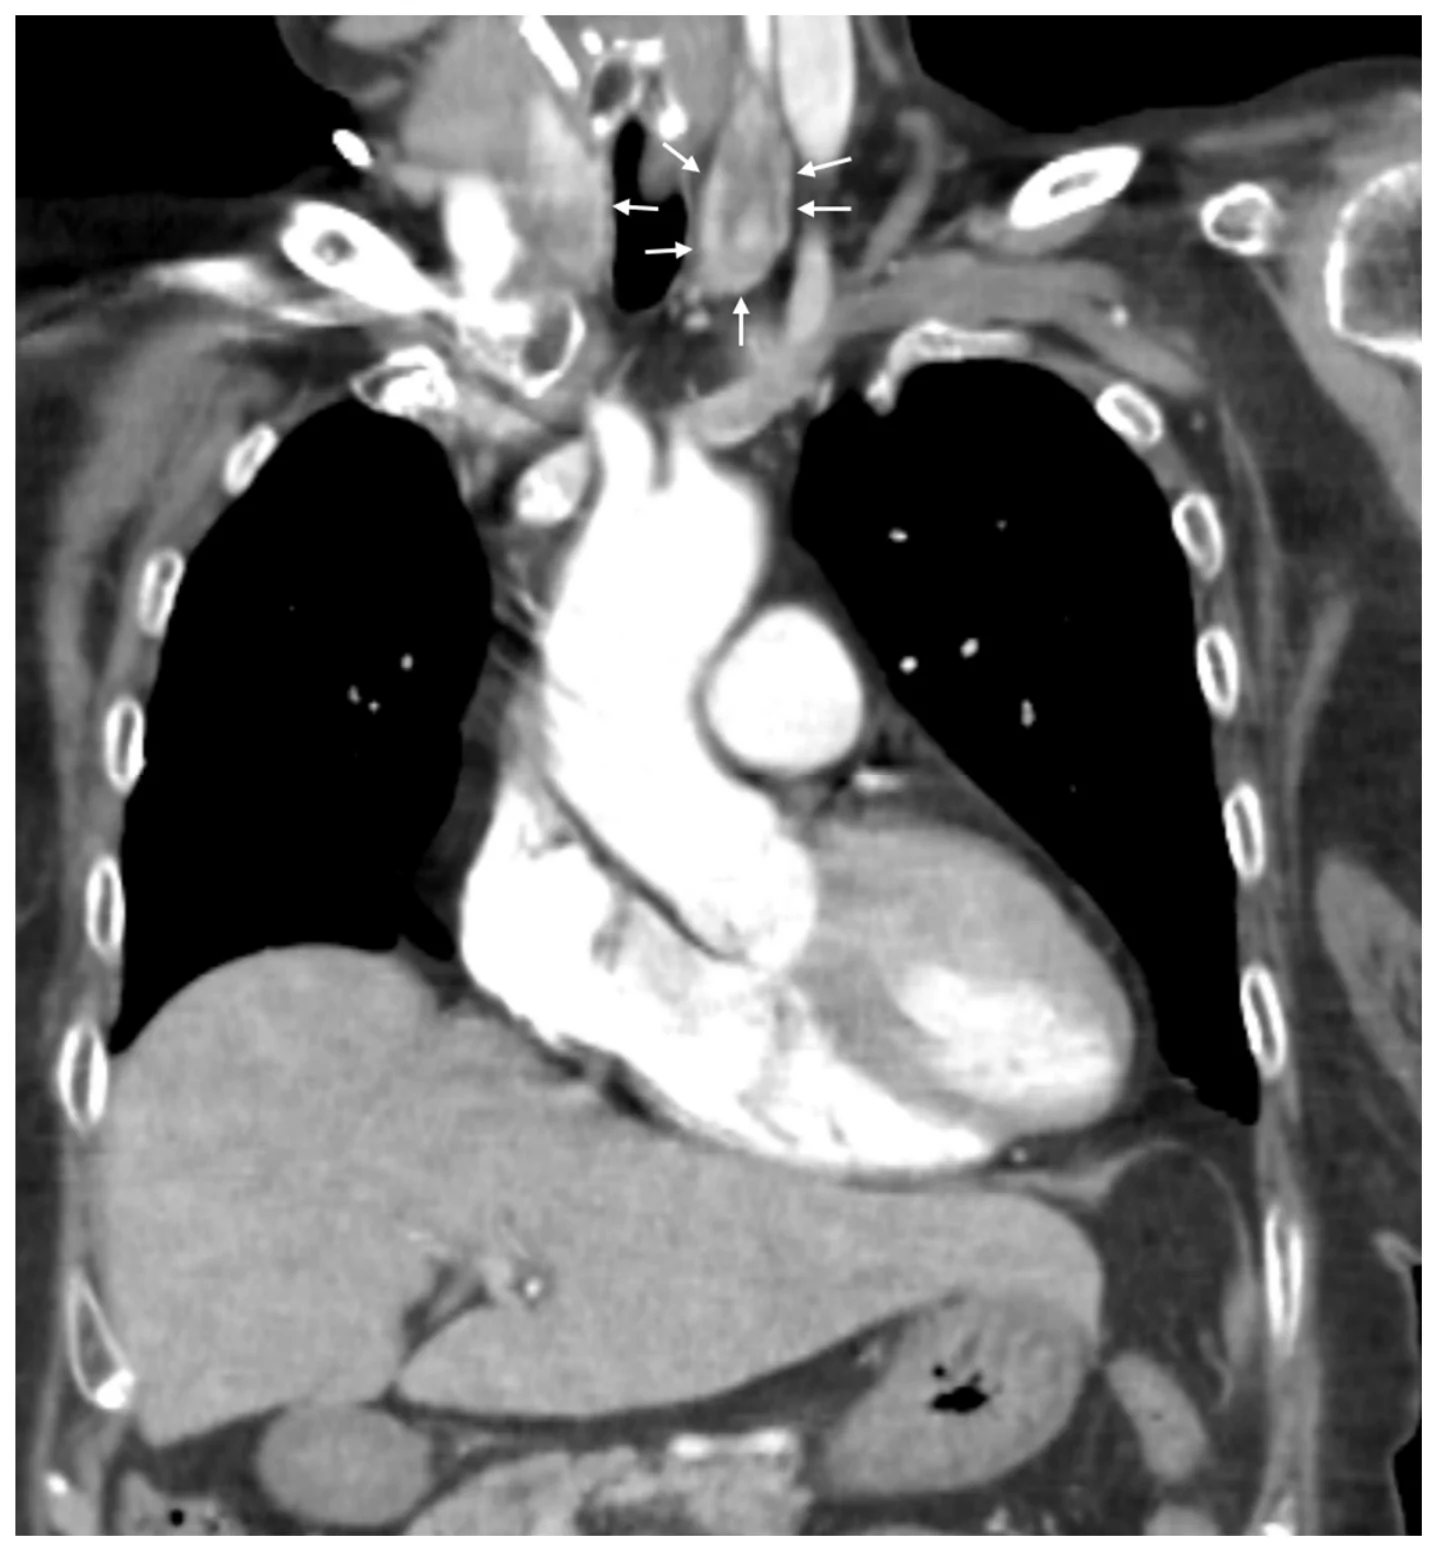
A case of probable shock thyroid in a 79-year-old woman. Note capsular linear sparing with low attenuation (arrows) in both thyroid glands. The enlarged thyroid glands show inhomogeneous enhancement. Thyroid function was normal in this patient on the same day as the CT.
